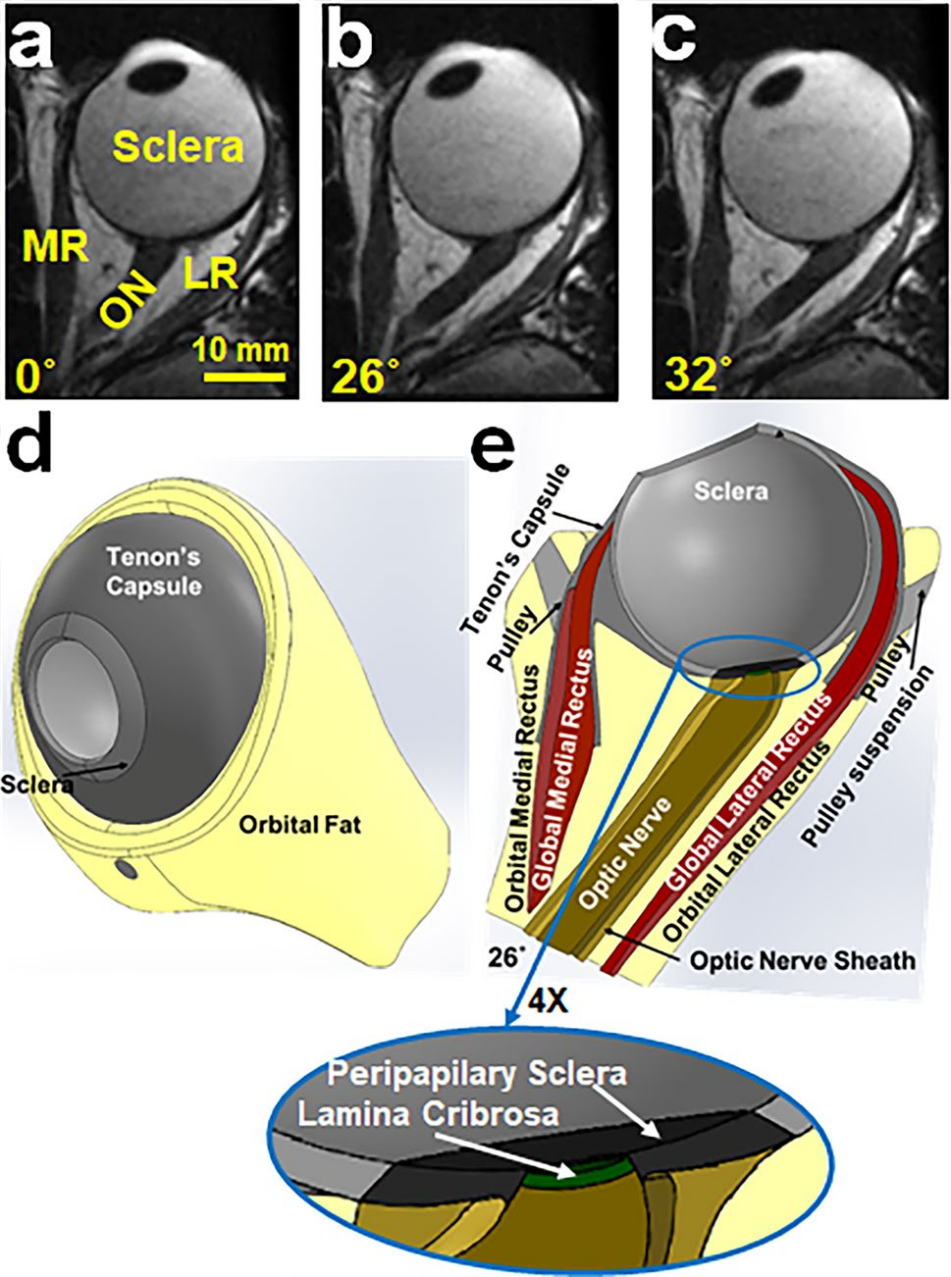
Axial magnetic resonance imaging (MRI) of a left orbit. In a central gaze 0°, where ON is sinuous. b 26° adduction where ON is first straight; and c 32° adduction where elongated ON remains straight. d 3D orbit surface rendered in SolidWorks, and horizontally hemisected in e showing configuration in 26° adduction
